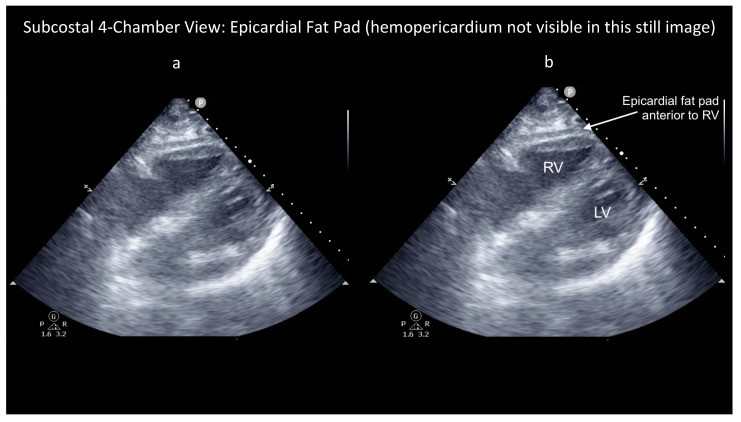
Unlabelled (a) and labelled (b) still image of a subcostal four-chamber (SC4C) view from a patient with concurrent epicardial fat pad and hemopericardium. This SC4C view shows a large epicardial fat pad anterior to the right ventricle (RV). Left ventricle (LV) also visible. Note that this patient’s concurrent pericardial effusion is not visible in this still image because it was only transiently visible in this view, specifically during ventricular systole (see Supplementary Video S3), but was readily apparent throughout the cardiac cycle in the parasternal long-axis view (see Figure 4 and Supplementary Video S4).
